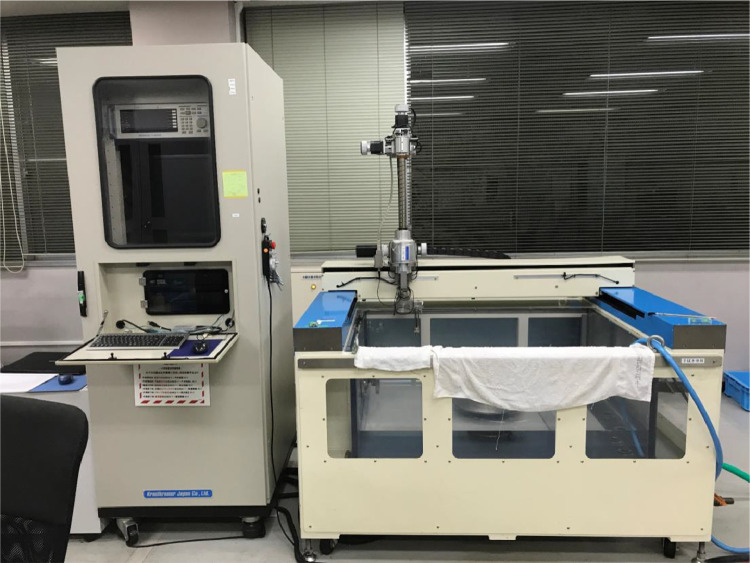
Ultrasound C-scanning system HIS3.
